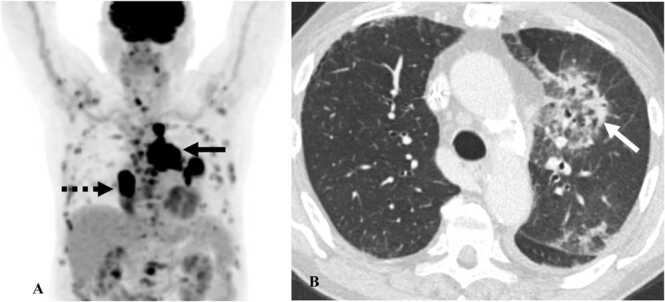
Immune-mediated pneumonitis in a 78-year-old man with small-cell lung cancer on Atezolizumab therapy. A. MIP image from PET/CT shows extensive hilar and mediastinal lymphadenopathy (black arrow) and right lower lobe lung mass (dashed black arrow). B. Contrast-enhanced axial chest CT image shows the development of new consolidation with central ground glass opacities (Atoll’s sign) in peribronchovascular distribution (white arrow) consistent with organizing pneumonia pattern of pneumonitis.
